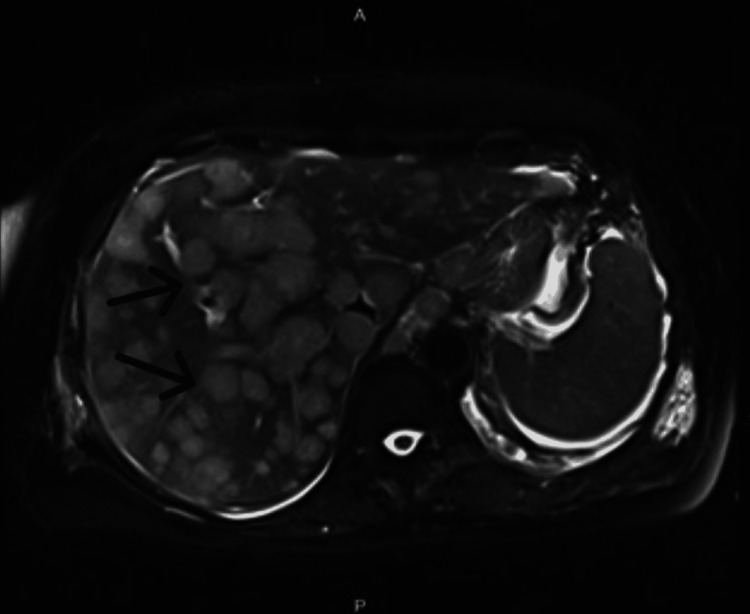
MRI of the abdomen Analysis reveals primary mass at the gastroesophageal junction (not shown) and also extensive metastatic disease to the liver (black arrows) with slight early ascites (not shown).
